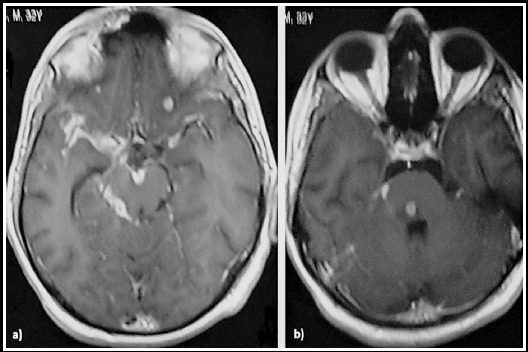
Axial Gadolinium-enhanced T1 weighted MRI images of 32 years old man show a) Meningeal enhancement that is more pronounced in the basal cisterns with small tuberculoma in the frontal lobe of the left cerebral hemisphere, b) Tuberculoma in the pons of the same patient with surrounding edema.
